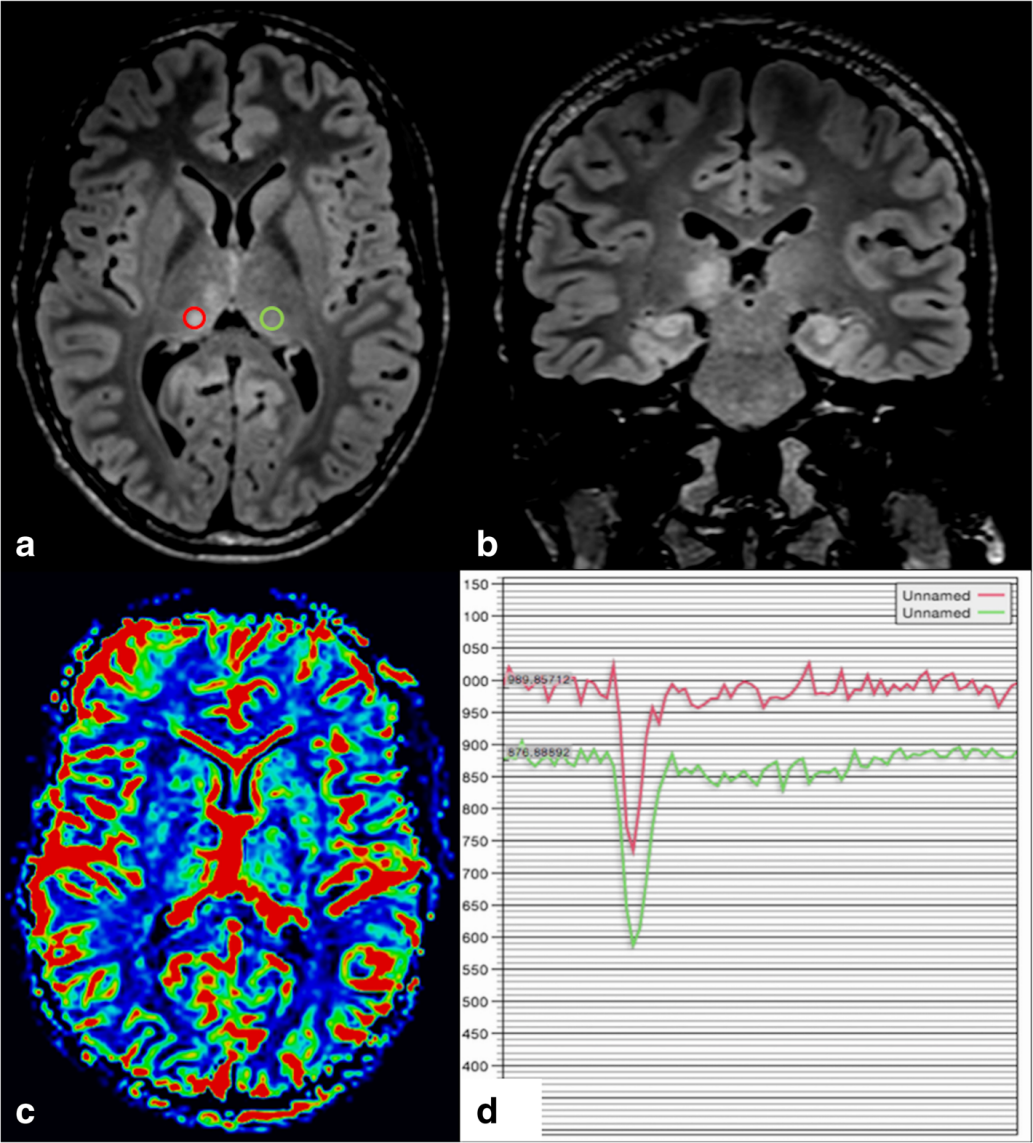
Bright confluent foci in the right thalamus on axial and coronal (a, b) FLAIR, not associated with mass effect or surrounding oedema. Absence of hypervascularisation on perfusion MR (b, c) further confirms the benign nature of these lesions allowing differentiation between primary brain tumour such as astrocytoma or glioblastoma and benign lesions. These are FASIs of a patient with NF1
